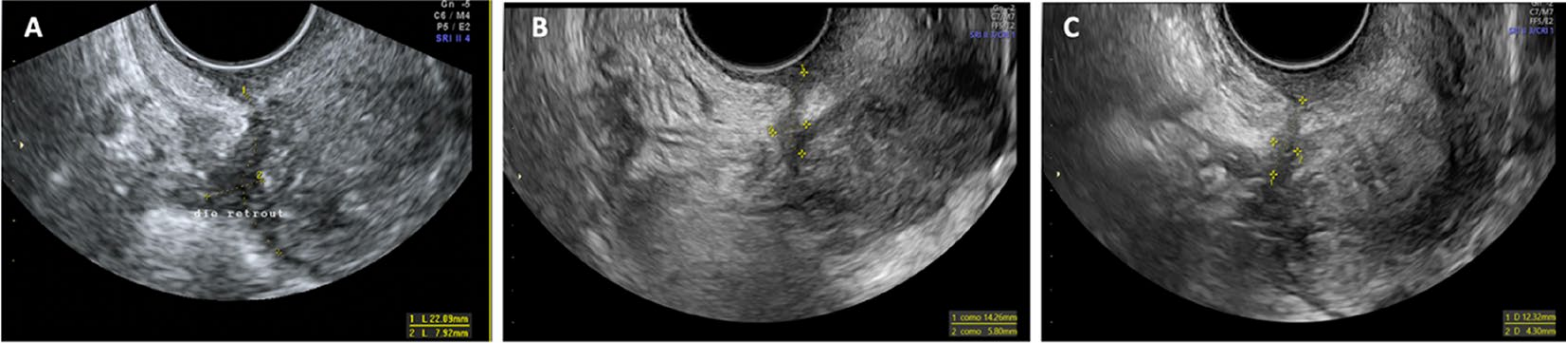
Sonographic evolution of uterosacral deep endometriosis at baseline and at the 12- and 24-month follow-ups. Image (A) Uterosacral deep infiltrating nodule with a mean size of 15.0 mm which decreased to 10.0 mm at the 12-month follow-up in image (B) and to 8.3 mm at the 24-month follow-up in image (C).
